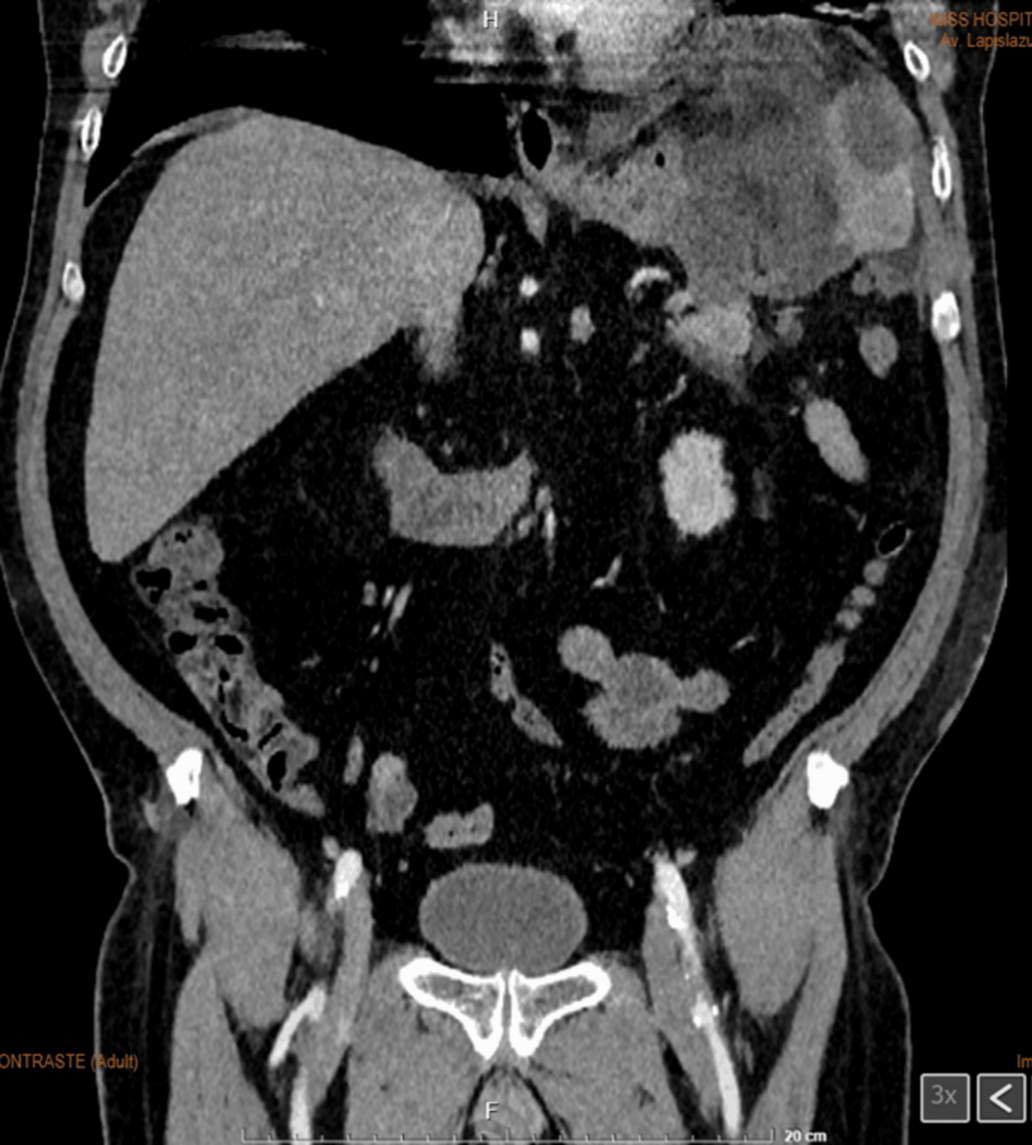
Contrast-enhanced abdominal computed tomography in coronal view showing a collection localized within the spleen with central density variation.
